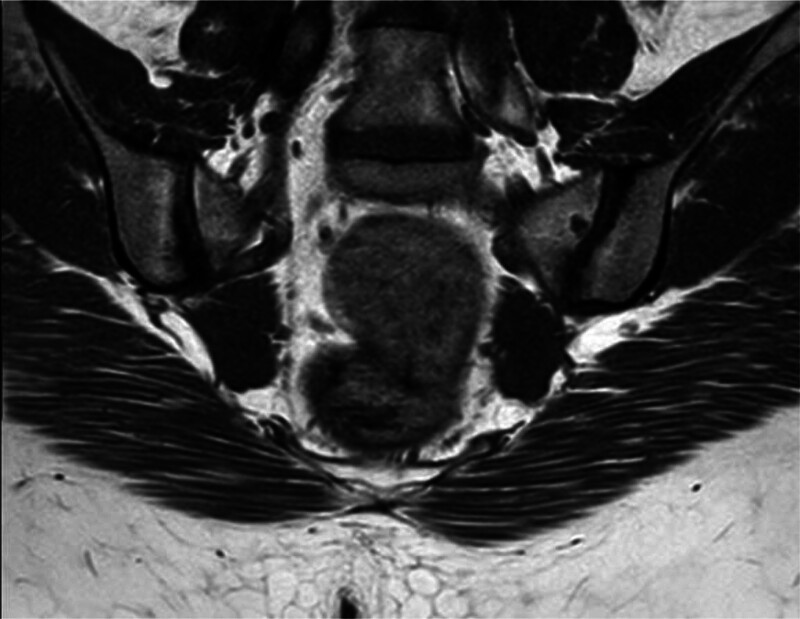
Axial T1-weighted MRI of the sacroiliac joints demonstrates bilateral subchondral sclerosis, with asymmetric fatty metaplasia more pronounced on the right, and a small right-sided subchondral cyst, consistent with chronic structural changes of sacroiliitis. MRI = magnetic resonance imaging.
